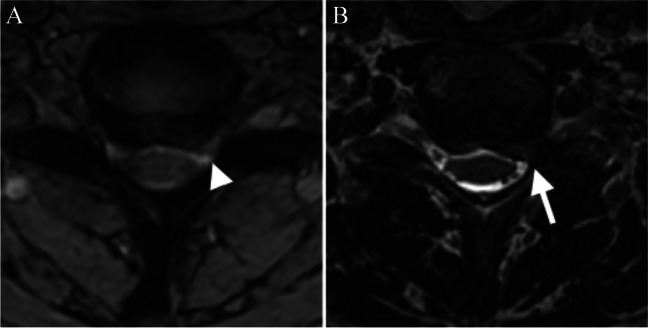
Comparison of 2D and 3D MRI sequences with corresponding axial images at the cervical level of C6/7. In the conventional gradient echo axial image, there seems to be only mild stenosis on the left side (white arrowhead) (A). In the 3D T2 TSE (SPACE) image, severe stenosis is detected (white arrow) (B)
